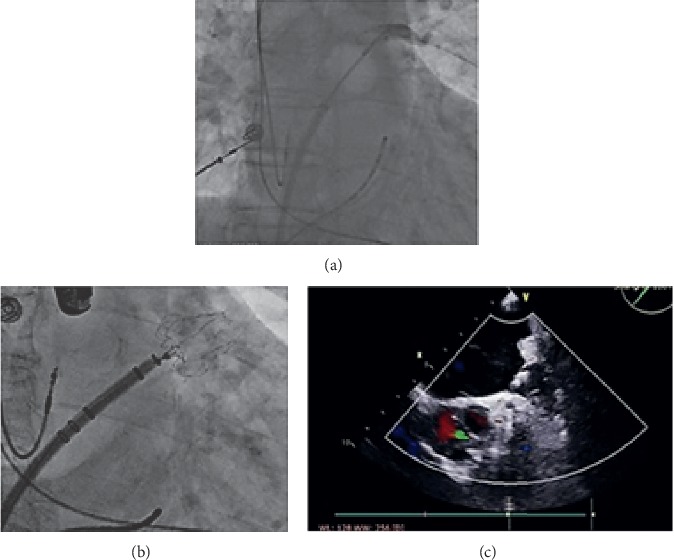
Procedure details about CBA and LAAC. (a) Balloon ablation of left superior pulmonary vein (LSPV) that fully occluded by angiography. (b) Deployment of the WATCHMAN device under fluoroscopy. (c) Transoesophageal echocardiography (TEE) showed complete occlusion without malposition or residual flow.
